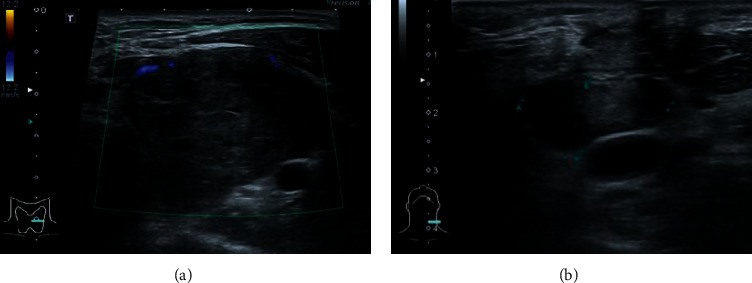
Ultrasound of the thyroid gland showing (a) a large heterogenic hypoechoic mass occupying the entire left thyroid lobe with no hypervascularity and (b) an enlarged left cervical lymph node with cystic necrosis.
